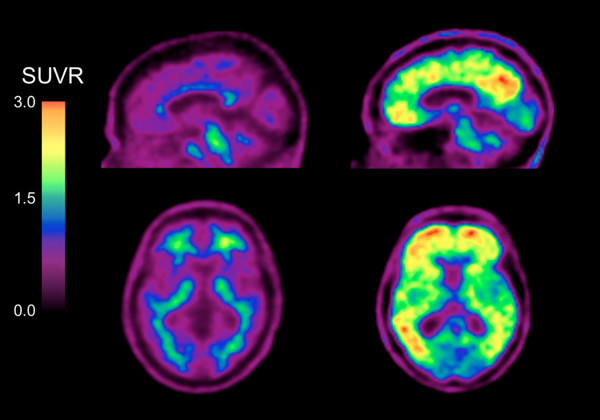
Sagittal and transaxial ^18^F-florbetaben positron emission tomography images of two mild cognitive impairment participants. Representative sagittal and transaxial ^18^F-florbetaben positron emission tomography (PET) images of two female mild cognitive impairment participants, both of the same age (73 years old) and with the same Mini-Mental State Examination score of 27. While the PET images on the left show nonspecific retention in white matter, the PET images on the right show high cortical ^18^F-florbetaben retention in the typical pattern seen in Alzheimer's disease, with highest retention in the precuneus/posterior cingulate, frontal and lateral temporal cortices. SUVR, standard uptake value ratio.
